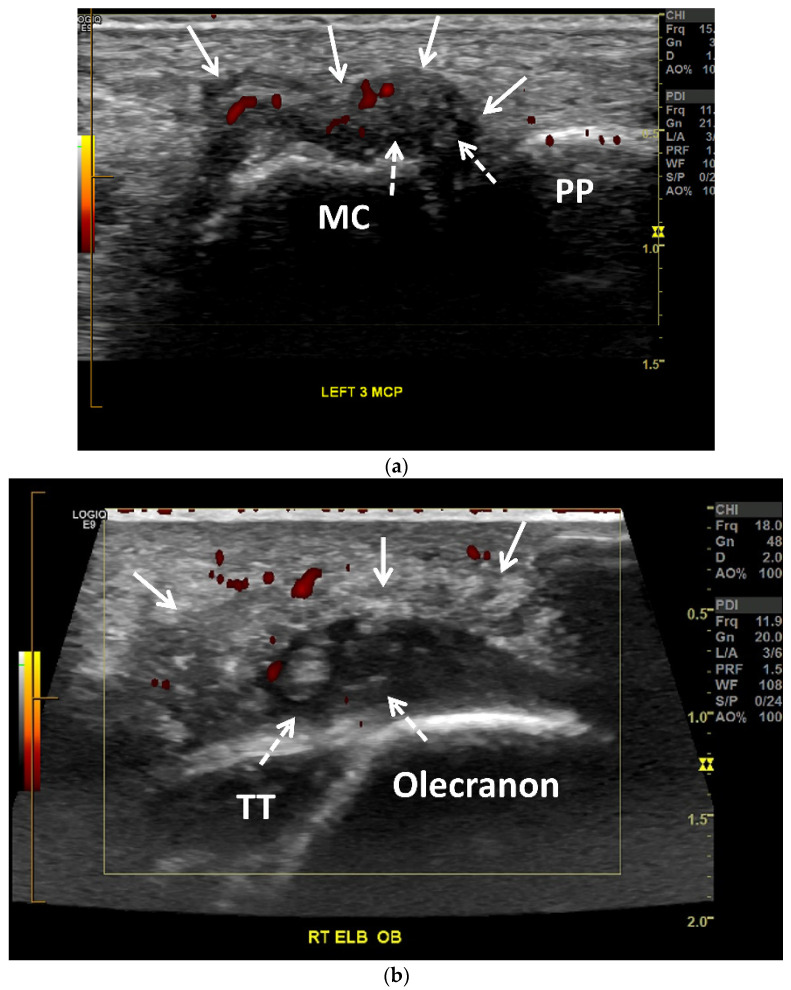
Gouty arthropathy in a 66-year-old woman. (a) Long-axis power Doppler US image along the dorsal aspect of the third metacarpophalangeal joint shows a moderate distension of the joint capsule (arrows) with numerous small echogenic foci related to MSU crystals (dashed arrows), creating “snowstorm” appearance. Note associated mild-to-moderate hyperemia (red). MC = metacarpal head. PP = proximal phalanx. (b) Obliquely oriented power Doppler US image along the posterior aspect of the right olecranon shows a heterogenous moderately distended olecranon bursa (arrows) with numerous small echogenic foci related to MSU crystals (dashed arrows). Note associated mild hyperemia (red). The distal triceps tendon (TT) is hypoechoic with scattered tiny hyperechoic foci consistent with tendinopathy and MSU crystal deposition.
